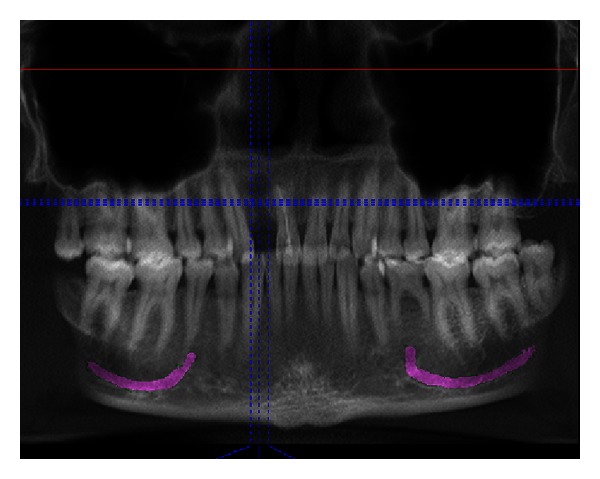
Preoperative CBCT with reformatted panoramic view. Gross radiolucency may be noted at the edentulous site.
